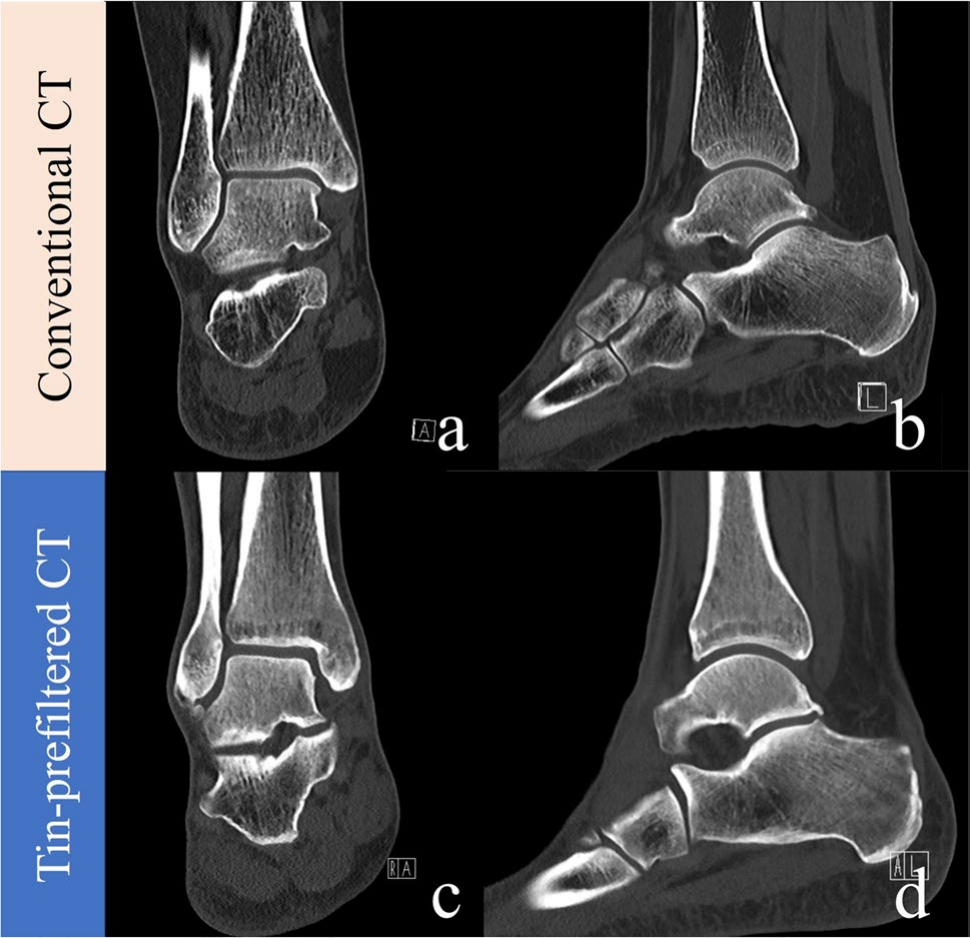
Conventional CT scan (a, b) of the ankle of a 38-year-old female (BMI 24.6 kg/m^2^) compared to a tin-prefiltered CT scan (c, d) of the ankle of a 39-year man (BMI 24.6 kg/m^2^). CTDI_vol_ of the tin-prefiltered scan was 3.07 mGy compared to 6.87 mGy in the conventional CT scan. All images were reconstructed with the convolution kernel BR62, iterative reconstruction strength level 3, and displayed at a window/center level of 1500/450. Both coronal images have a slice thickness of 1 mm, and both sagittal images have a slice thickness of 2 mm
